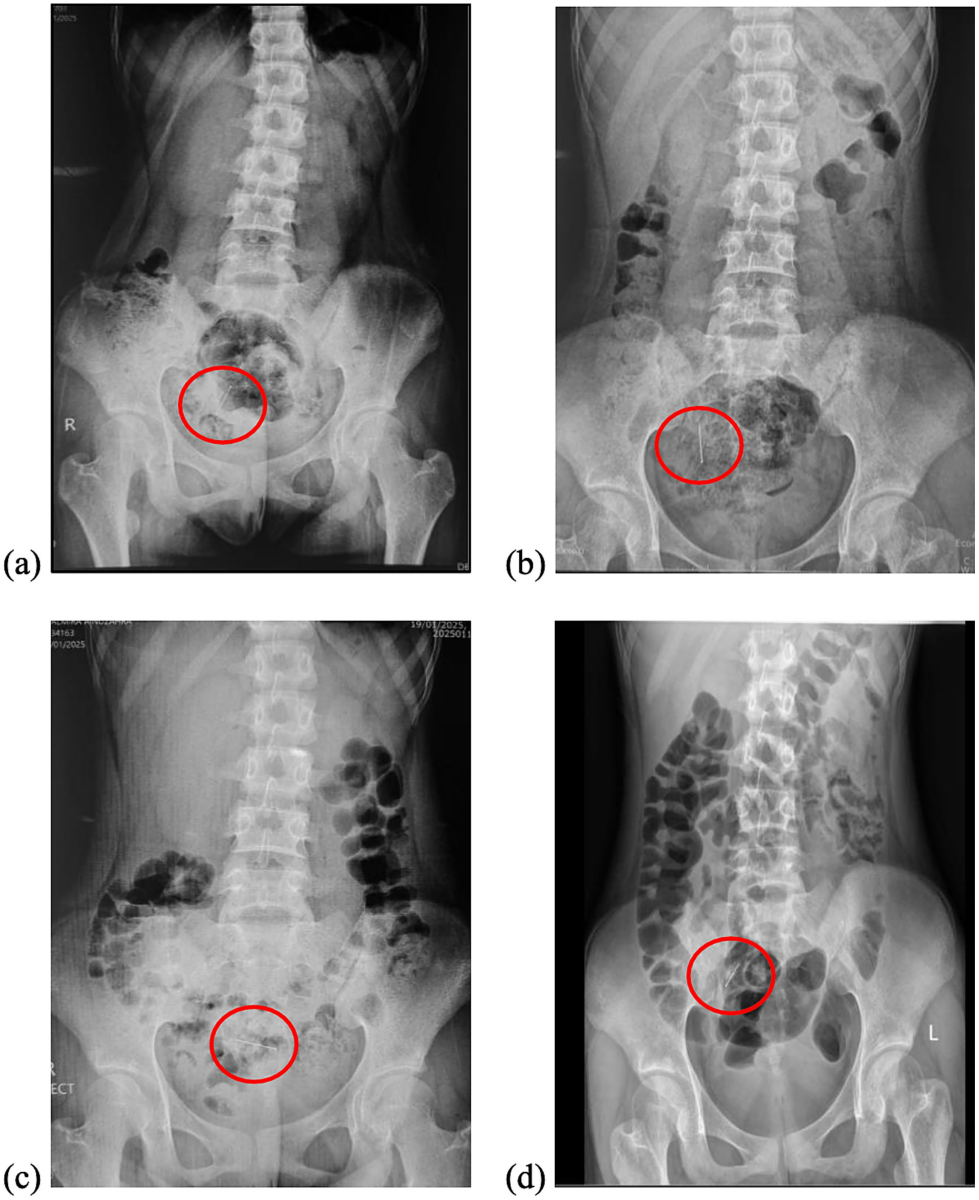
Serial abdominal radiographs demonstrated the presence of a pin (inside the red circle) in the lower quadrant of the abdomen with no acute findings. (a) Day of the ingestion (Day 0), (b) Day 3, (c) Day 7, and (d) Day 21.
